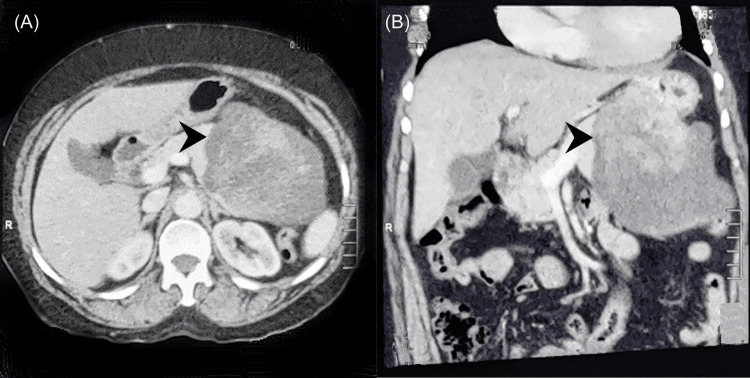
Contrast-enhanced Computed tomography of the abdomen of one patient. (A) axial section (B) coronal section showing large well-defined solid cystic mass with areas of hemorrhage in the tail of the pancreas (arrowhead).
